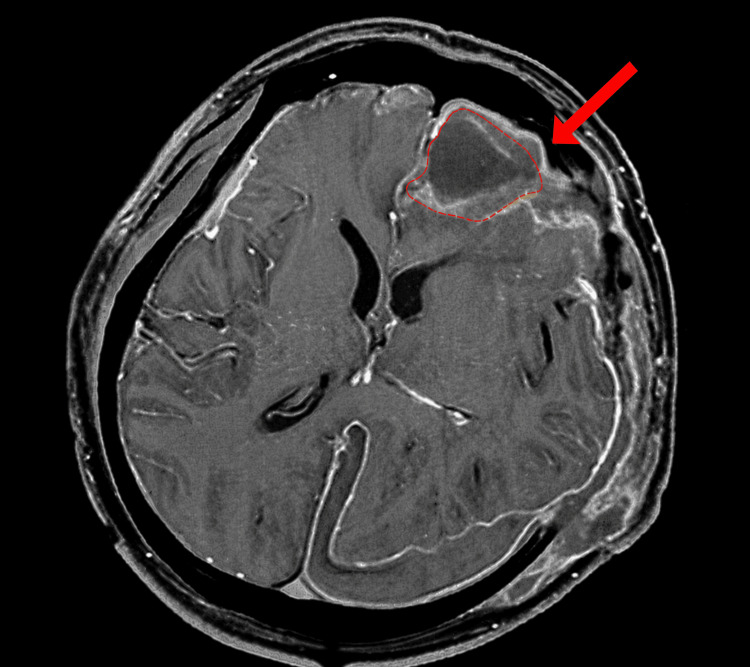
Axial post-contrast T1-weighted MRI of the brain. The red arrow and dashed outline highlight the heterogeneously contrast-enhancing lesion in the left frontal lobe.
